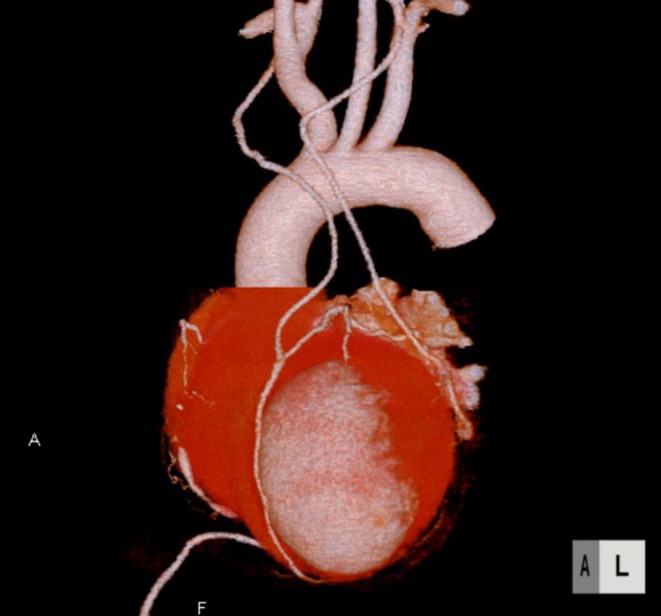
Postoperative computed tomography showing patency of all grafts.
